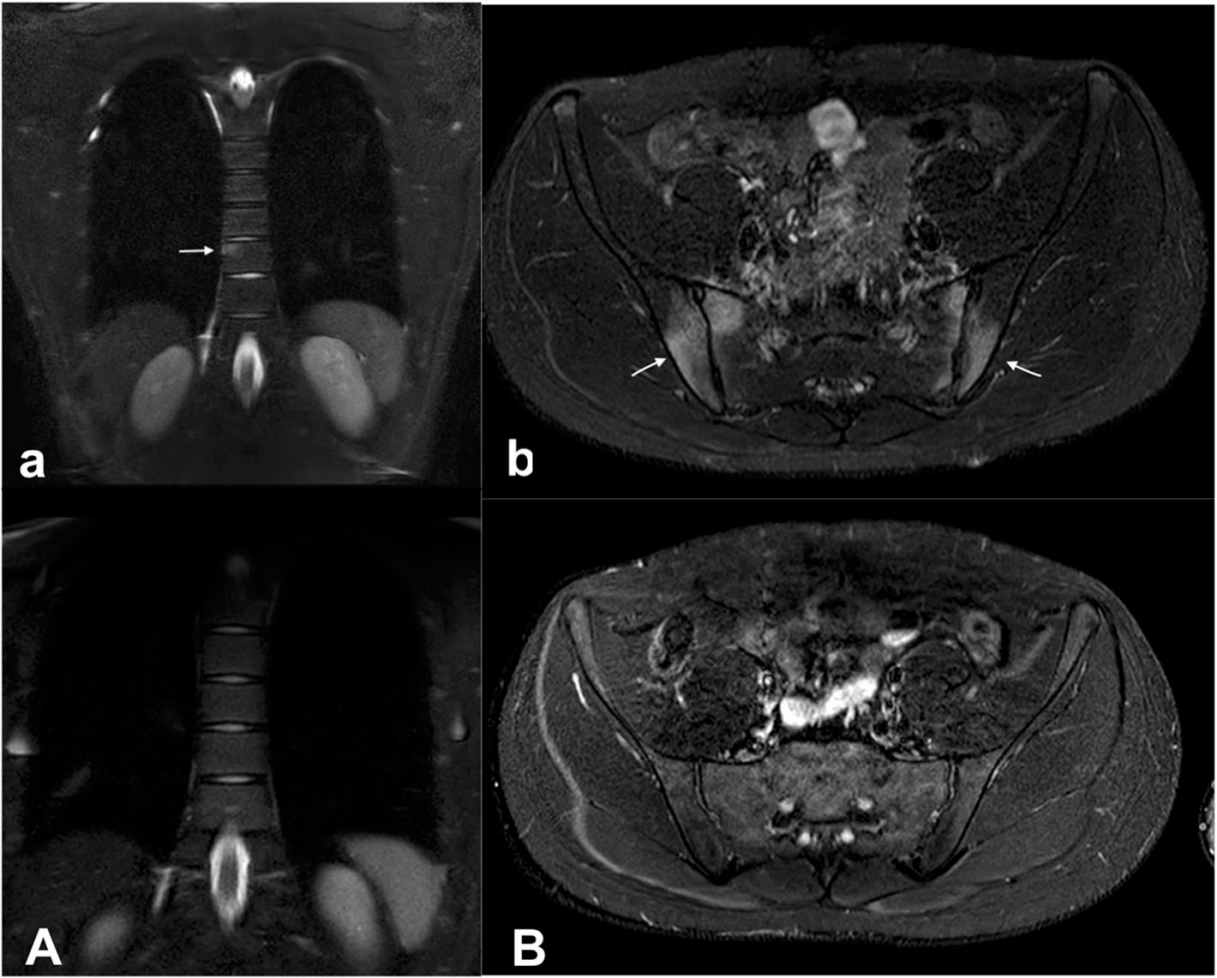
MRI STIR images of lumbar osteitis and sacroiliac involvement before (upside) and after ADA treatment (downside)
